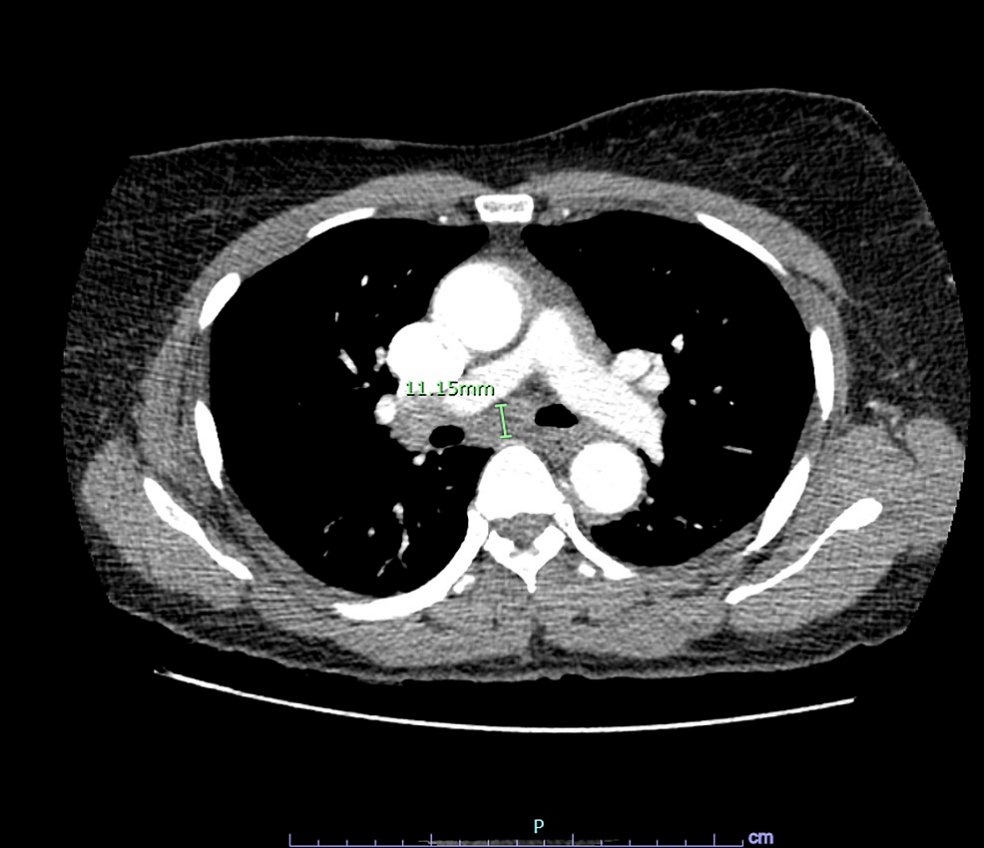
CTA image of lymphadenopathy near the carina of 1.1 cm CTA: computed tomography angiography
